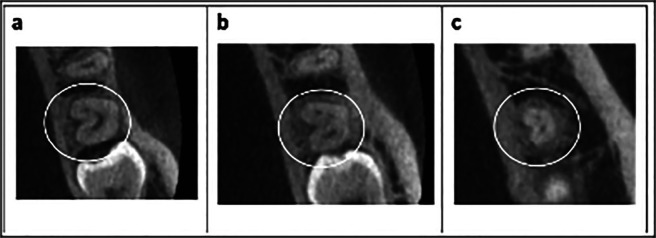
CBCT images of a molar presenting different configurations according to third: (a) C1 in the coronal third, (b) C3a in the middle third, and (c) C3b in the apical third.
